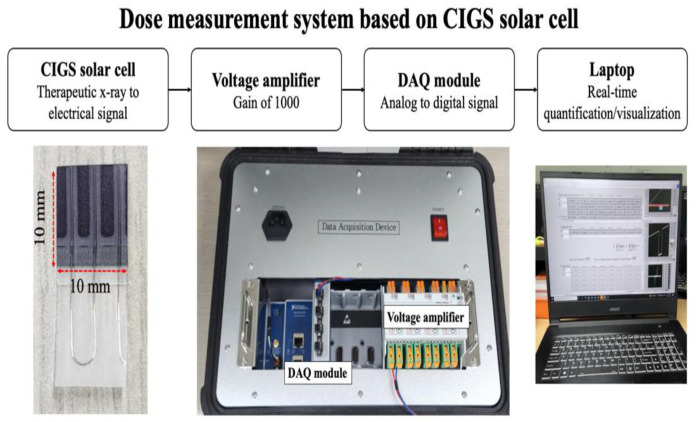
Configuration and data acquisition process of our measurement system based on a copper indium gallium selenide (CIGS) solar cell. In this system, therapeutic X-ray radiation is converted to electrical signals by the CIGS solar cell, and the electrical signals were digitalized through data acquisition (DAQ) module. The digitalized signals were visualized in real-time using a laptop with in-house software.
